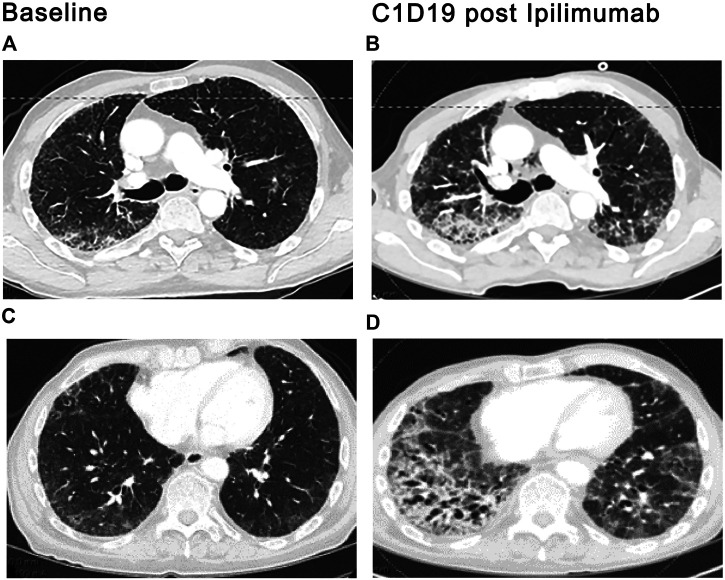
ICI-related sarcoid-like reaction. Diffuse lymphadenopathy in paratracheal, bi-hilar, and mediastinal locations (arrows), (A,C), developed after the third cycle of ipilimumab plus nivolumab therapy for bladder cancer. Diffuse interstitial and nodular infiltrates along bronchovascular bundles (arrows), (B,D) were also seen. Transbronchial biopsies and biopsies of the lymph nodes by endobronchial ultrasound (EBUS) demonstrated noncaseating granulomas. The diagnosis of ICI induced sarcoid-like reaction was confirmed once competing diagnoses were excluded.
